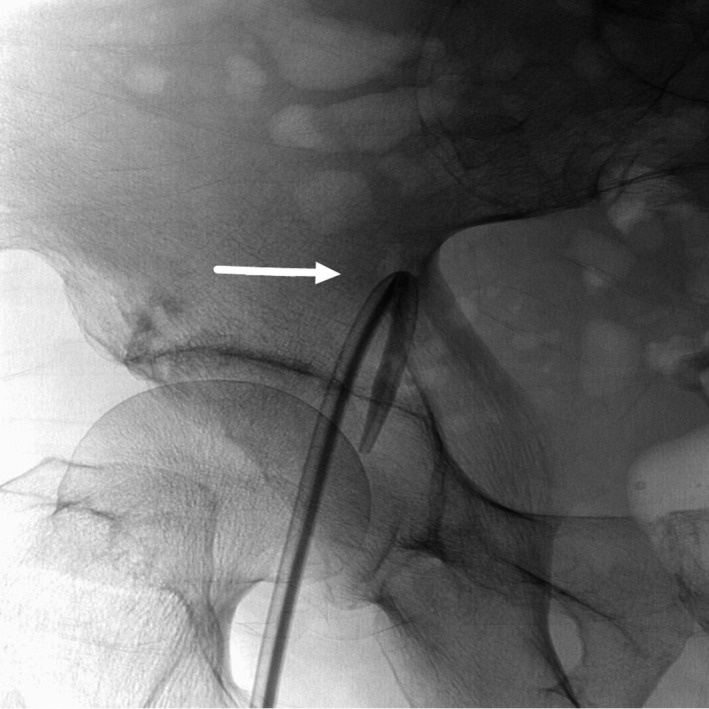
Radiography showing the curved and fractured catheter in the right femoral and iliac veins (arrow).
